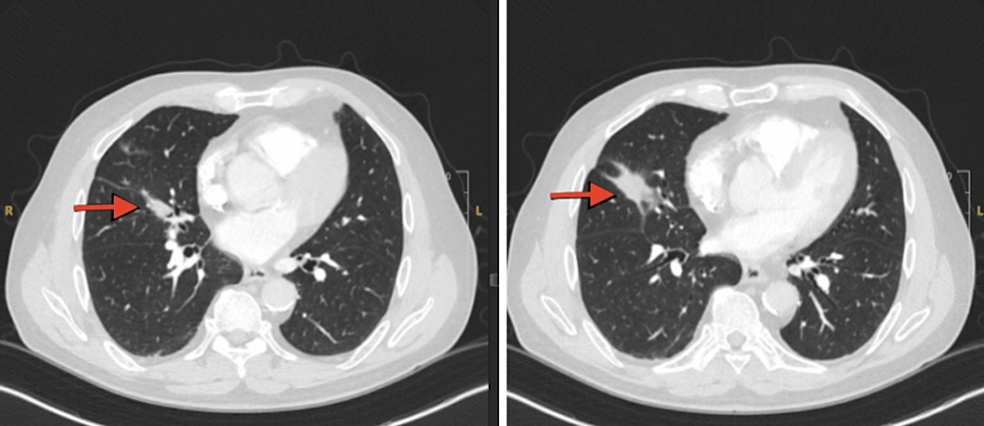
CT of the chest images showing evidence of right middle lobe endobronchial lesion (red arrowheads) resulting in focal post-obstructive collapse
